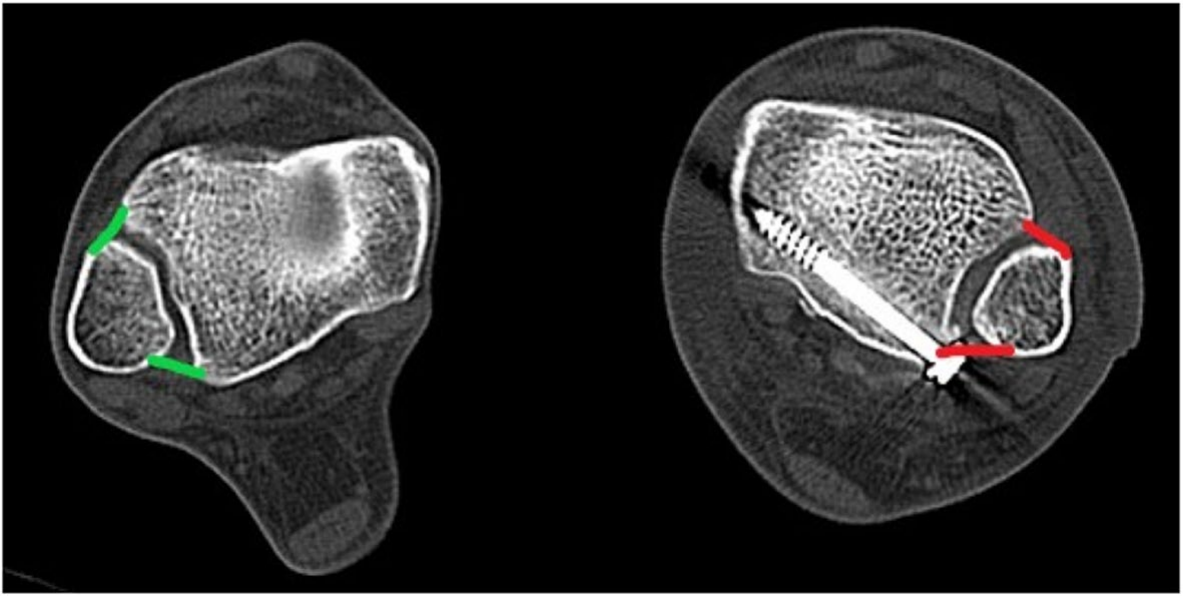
A difference and P difference were calculated as the distance between the anterior and posterior facets of the tibial incisura and the fibula. Notes: This patient underwent the AITFL anatomical repair technique. The operative side (red) is compared to the uninjured side (green) on computed tomography (CT) at 6 months postoperatively
